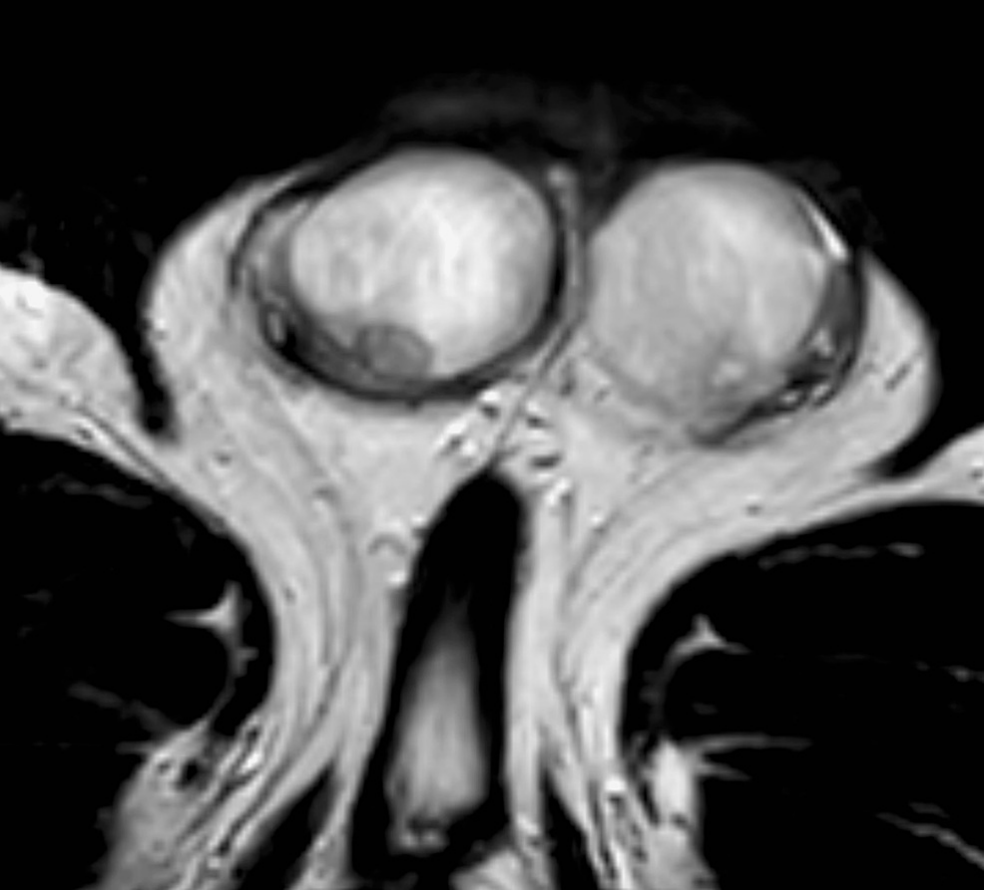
T2-weighted MRI of the pelvis at two-month follow up Transversal image showing significant reduction of both number and size of testicular lesions
